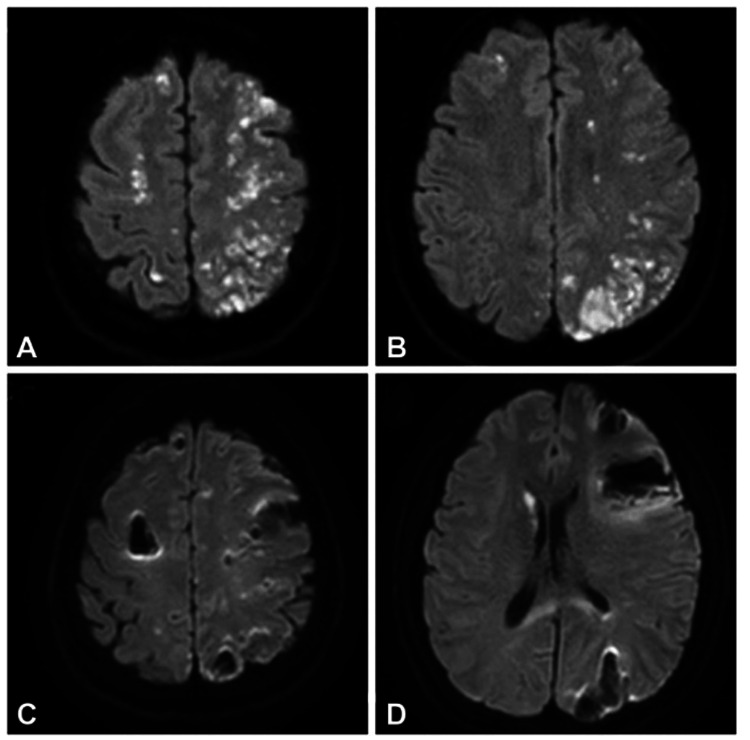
MRI brain image showing acute embolic infarction after facial hyaluronic acid filler injection into the glabella area (A, B) Brain diffusion MRI on day 1 after facial filler injection showing numerous high signal-intensity lesions in both cerebral hemispheres, involving the frontal, parietal, and occipital lobes. These were acute embolic infarcts caused by filler-related emboli. (C, D) In follow-up diffusion MRI on day 7, extensive hemorrhagic transformation that could be categorized as parenchymal hematoma was noted in the previous infarcted foci, especially in both frontal and left parieto-occipital lobes. Image credit: Lee et al., 2021 [27] with permission.
